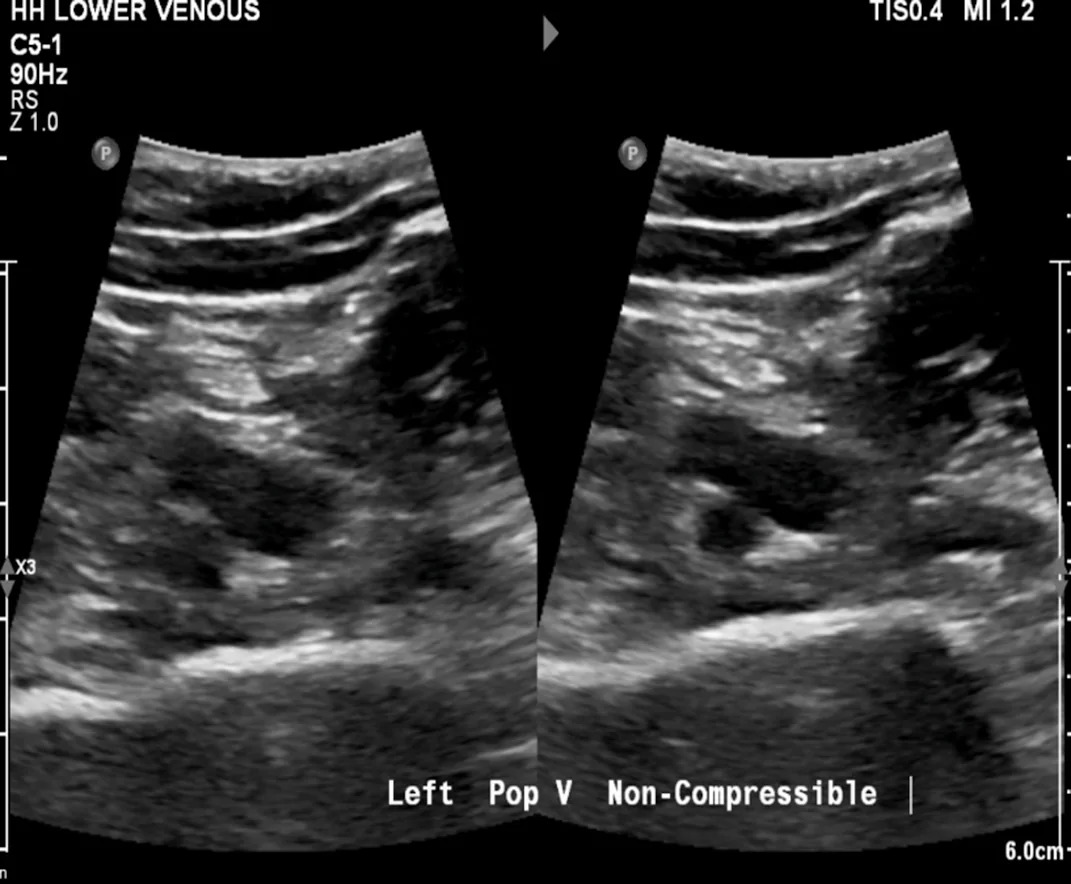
Venous Duplex Ultrasonography Demonstrating Acute Noncompressible Deep Vein Thrombosis Within the Popliteal Vein
